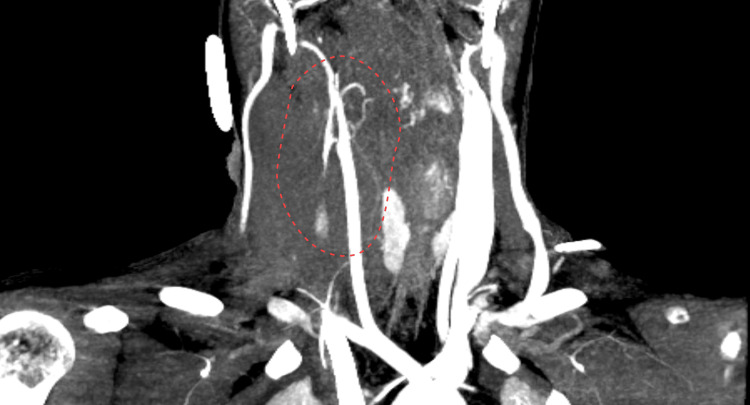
The coronal CT scan New collateral vessels in right side of the neck are partially shown.
